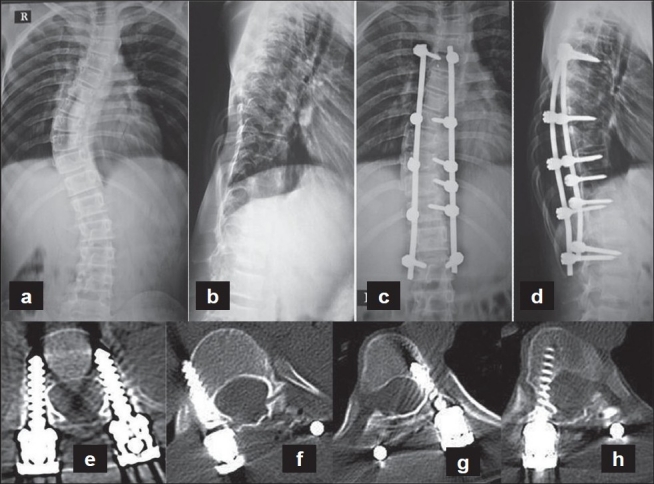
(a-d) Preoperative and post operative radiographs of an adolescent idiopathic right thoracic scoliosis. Computer assisted pedicle screw insertion was performed (e-h). Postoperative axial CT scan sections of the patient showing the placement of the screws
